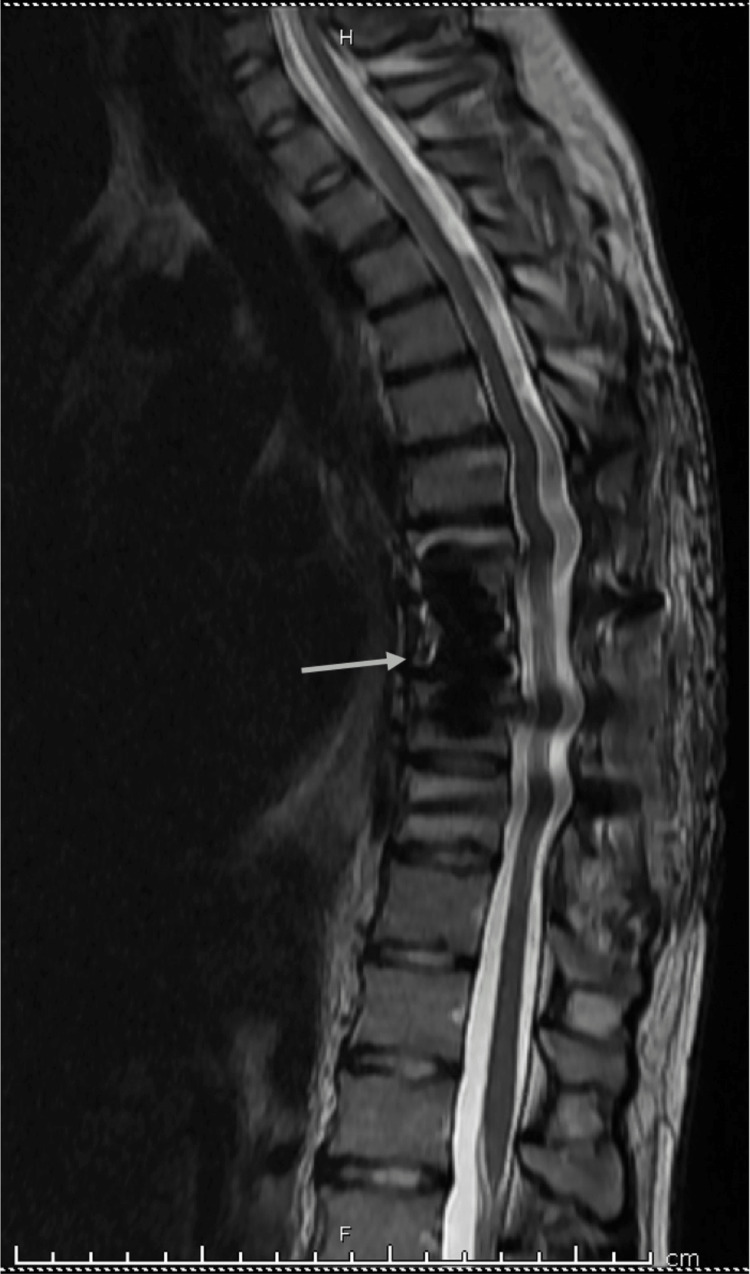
Six-month post-operative T2-weighted MRI demonstrating appropriate hardware fixation with no evidence of recurrence of the vertebral body mass or spinal cord compression.
